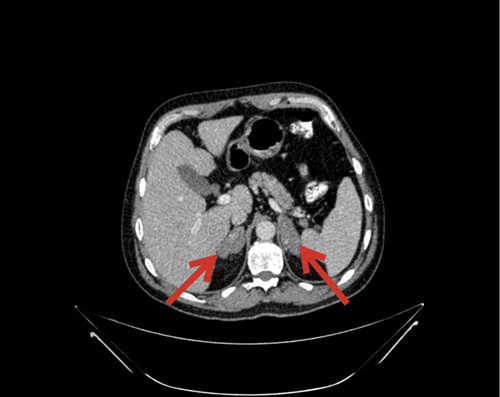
CT scan of the abdomen showing hepatosplenomegaly with bilateral adrenal hyperplasia.
